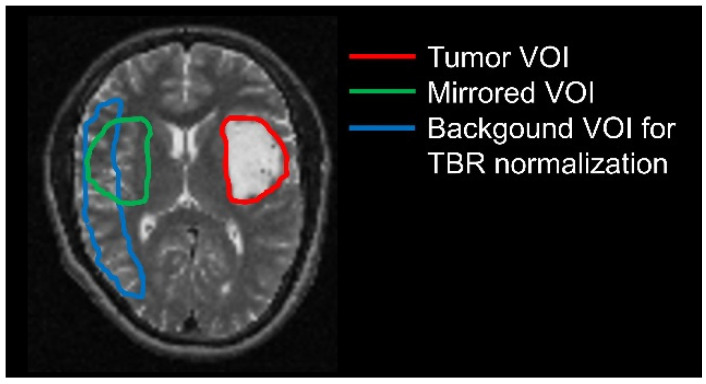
Volumes-of-interest used for analyses of [^18^]FET-negative gliomas: tumor VOI defined manually within T2/FLAIR images (red); tumor VOI mirrored to the contralateral site (green); background VOI for image normalization (blue).
